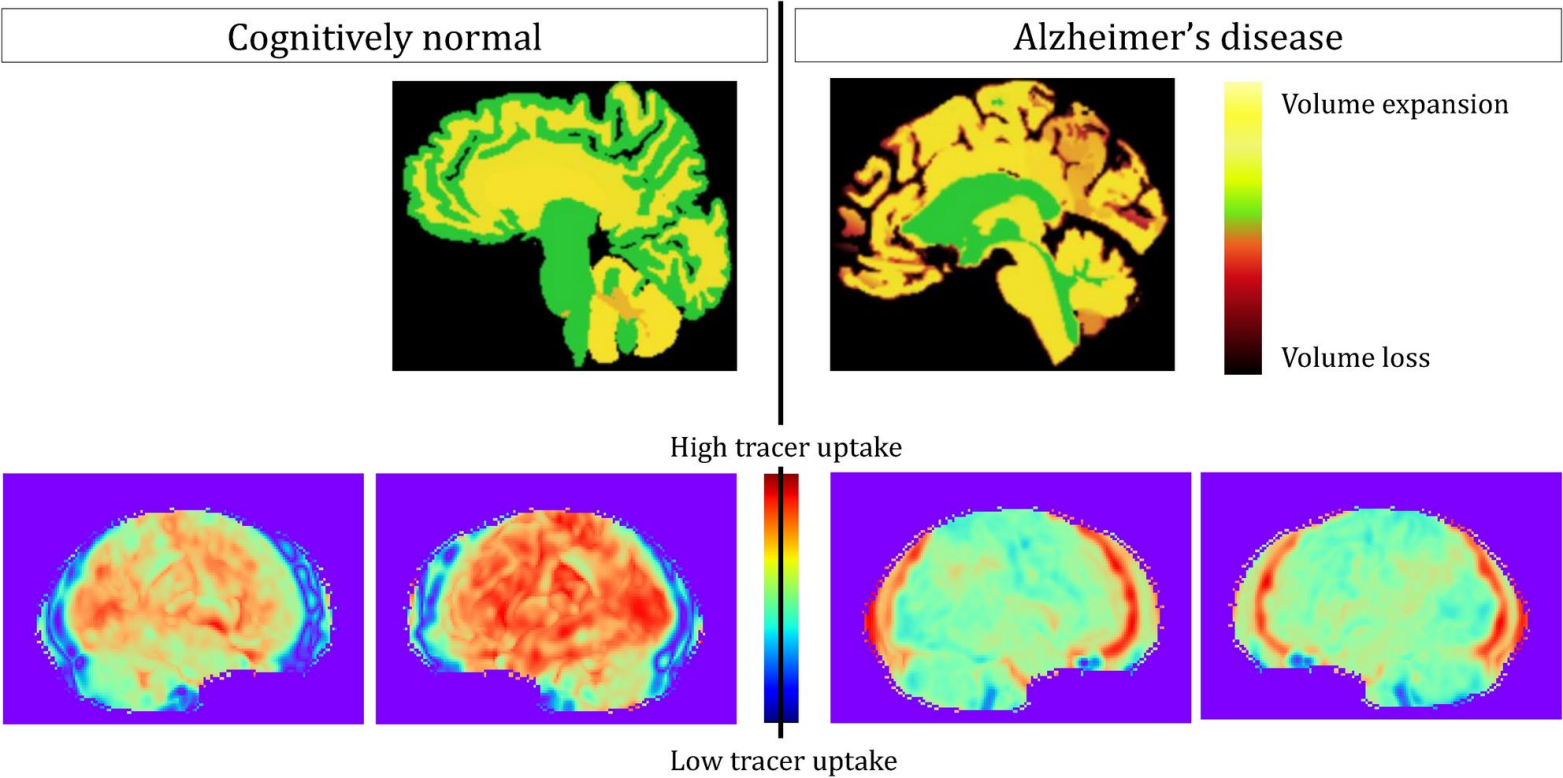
Sample MRI slices (top) and left lateral and right lateral 3D stereotactical surface projections (SSP) of FDG PET (bottom). For presentation purposes, the sample sagittal MRI slices (top) are colour-coded to show volumetric changes compared to 20 cognitively normal control participants. The left lateral and right lateral SSP of FDG PET scans (bottom) are colour-coded to show Fluorodeoxyglucose tracer uptake. The MRI slices are regenerated from^36^ with the authors’ permission.
